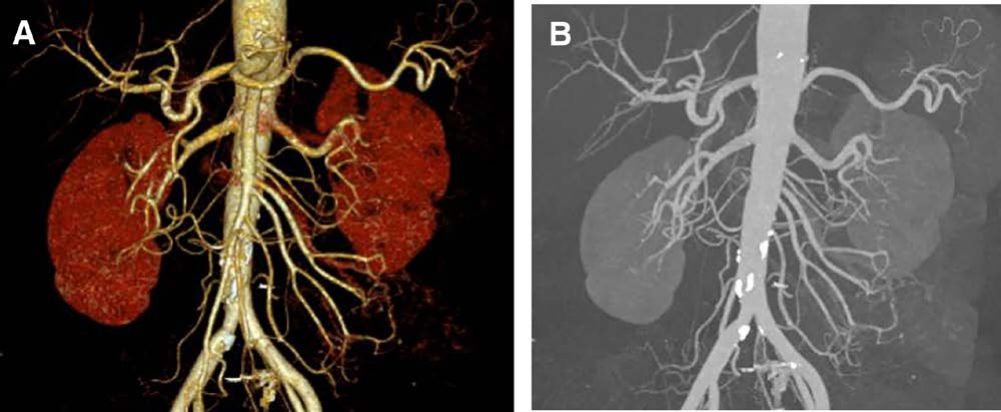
(a and b) Post-treatment CTA indicates substantial disappearance of nodules.
